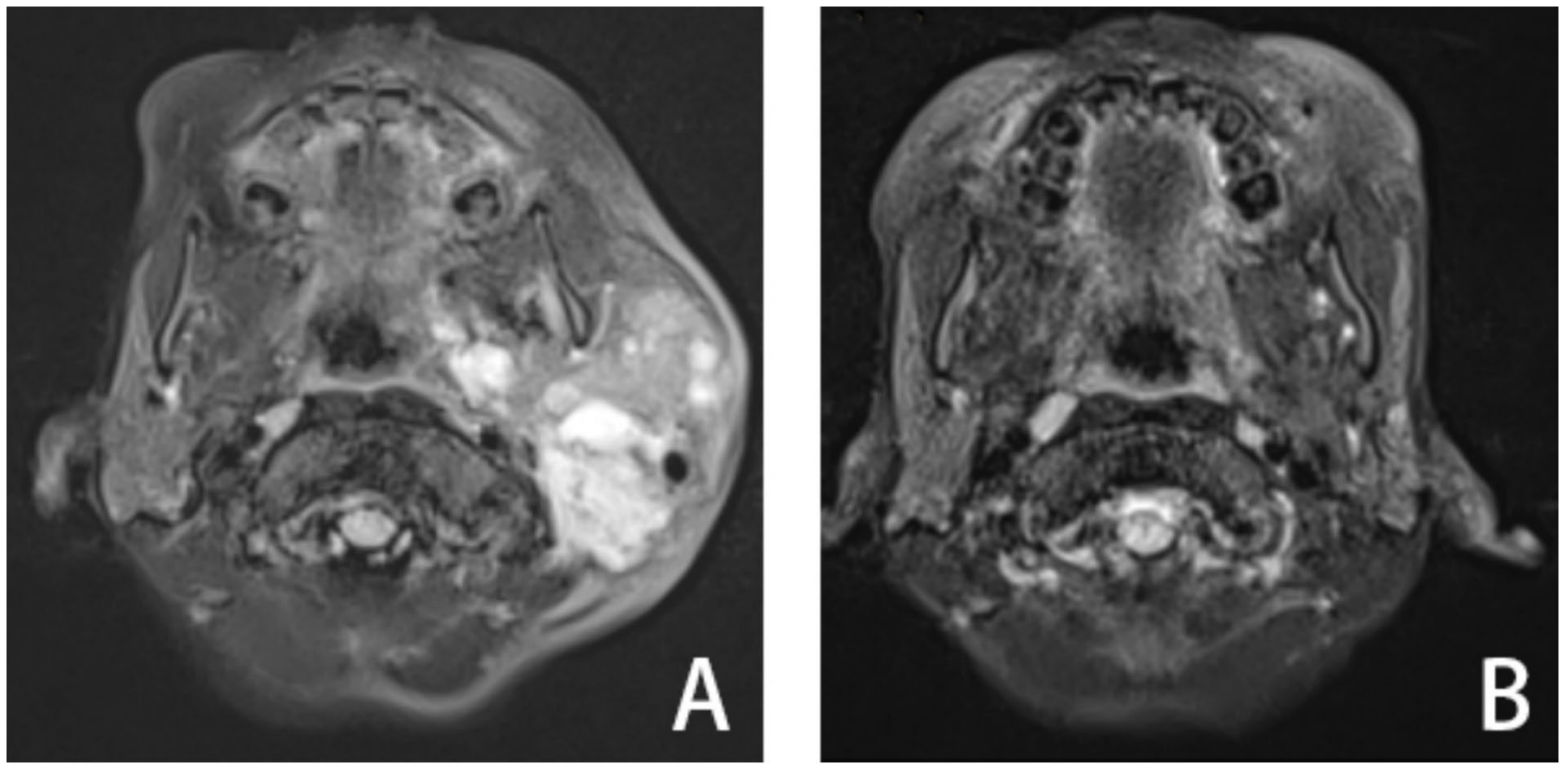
A patient with LM in maxillofacial region of T2-MRI axial scan: before treatment (A), 1 year after treatment (B).
